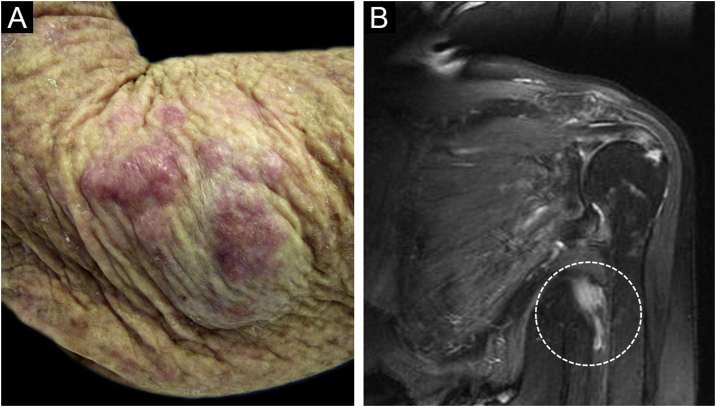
(A) Shiny pink nodules and papules in the left elbow area, representing and in-transit metastases; (B) Magnetic resonance imaging showing a nodular image next to the left axillary vascular-nervous bundle, suggestive of neoplasm.
